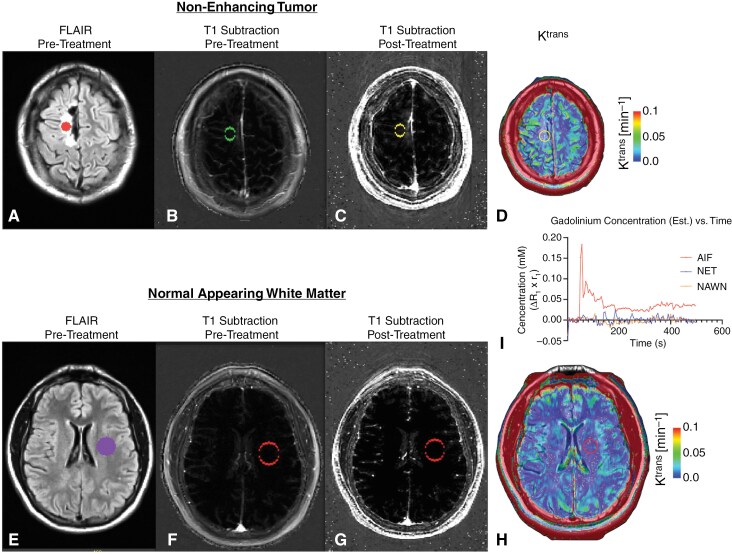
Example of T1 subtraction and DCE results after administration of regadenoson. (A) pretreatment FLAIR images showing region of interest (ROI) selection in areas of T2 hyperintensity consistent with non-enhancing tumor (NET). (B) pretreatment T1-weighted digital subtraction maps, created by subtracting post-contrast T1-weighted images from pre-contrast T1-weighted images, in areas of non-enhancing tumor. (C) Post-treatment T1-weighted digital subtraction maps in areas of non-enhancing tumor. (D) Post-treatment dynamic contrast-enhanced (DCE) perfusion magnetic resonance imaging (MRI) estimates of K^trans^, the forward flux or rate of contrast extravasation, and a surrogate of vascular permeability, in areas of non-enhancing tumor. (E) pretreatment FLAIR images showing ROI selection in areas of normal-appearing white matter (NAWM). (F) pretreatment T1-weighted digital subtraction maps in areas of NAWM. (G) Post-treatment T1-weighted digital subtraction maps in areas of NAWM. (H) Post-treatment DCE perfusion MRI estimates of K^trans^ in areas of NAWM. Estimated contrast agent concentration versus time for the arterial input function (AIF), ROI within the NET, and ROI within NAWM. Areas of NET and NAWM show almost no change in concentration after treatment.
